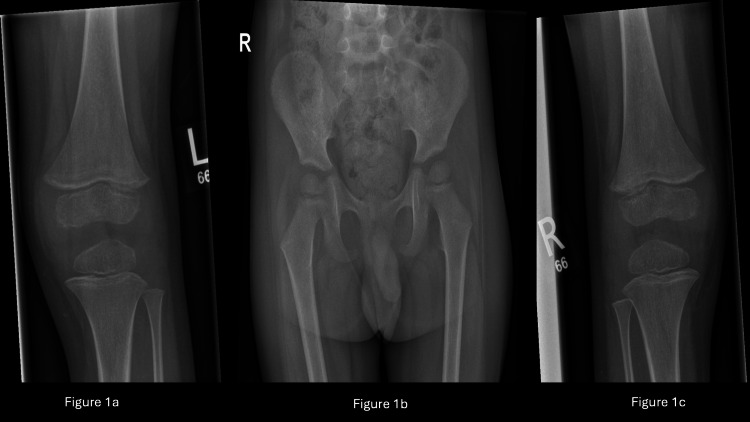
X-rays of pelvis and bilateral knees during initial presentation to Emergency Department Figure 1a is the left knee, Figure 1b is the pelvis and Figure 1c is the right knee. No fractures or bone lesions were observed. The hip joints appear symmetrical and no effusion is present in the knee joints.
